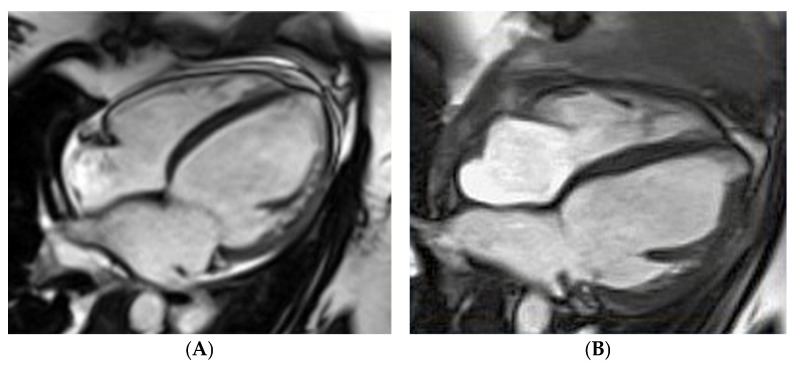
Peripartum cardiomyopathy in a gravida para26-year-old woman in cardiac magnetic resonance imaging: (A) fourth day postpartum, left ventricular ejection fraction (LVEF) of 17%, (B) after bi-ventricle assist device treatment, LVEF of 35% [18].
